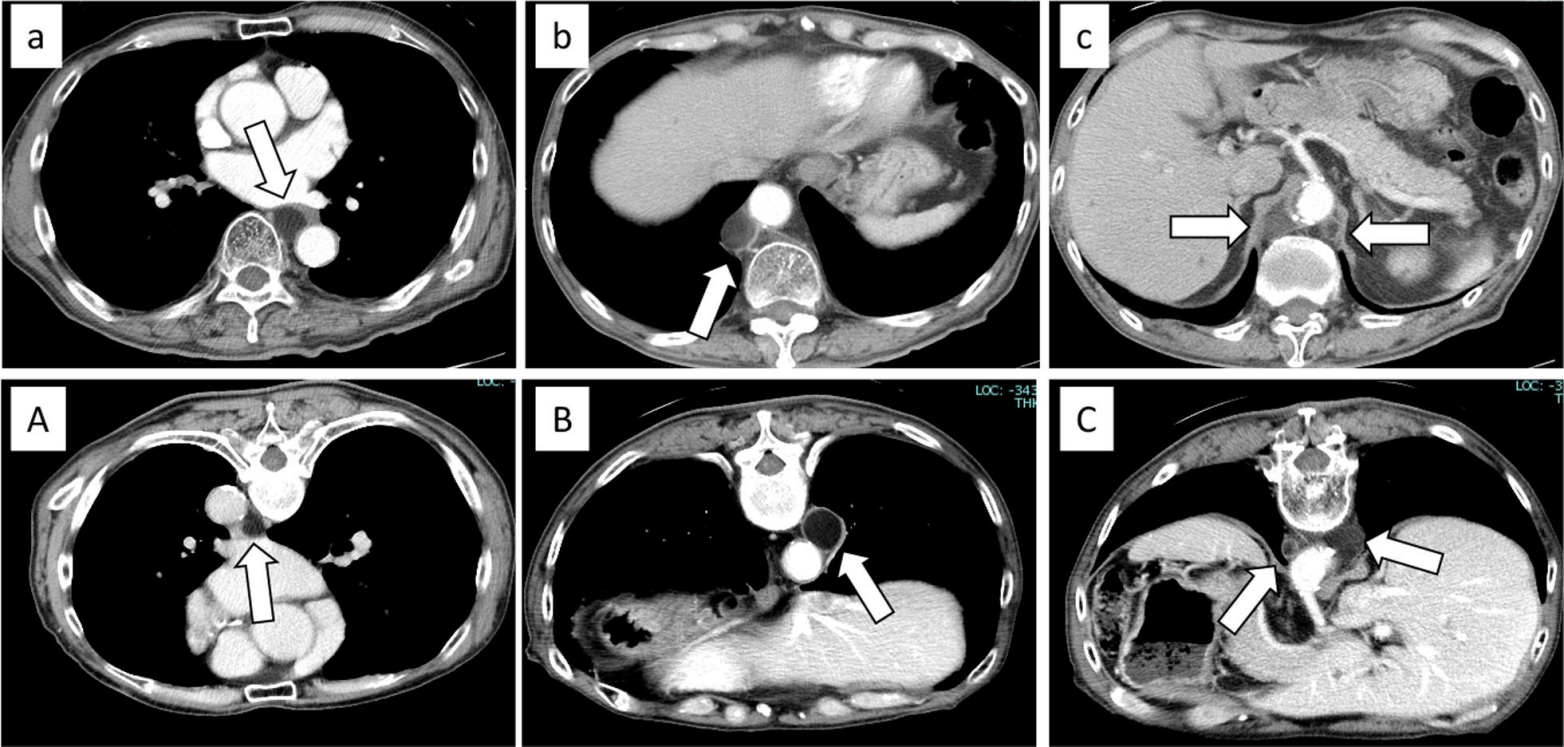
Contrast-enhanced computed tomography in the supine position in the upper row and in the prone position in the lower row. Comparison of a, A the most cephalic side of the tumor, b, B confluence of the hepatic vein and inferior vena cava, and c, C level at which the celiac artery branches off the aorta (white arrow: thoracic duct cyst)
